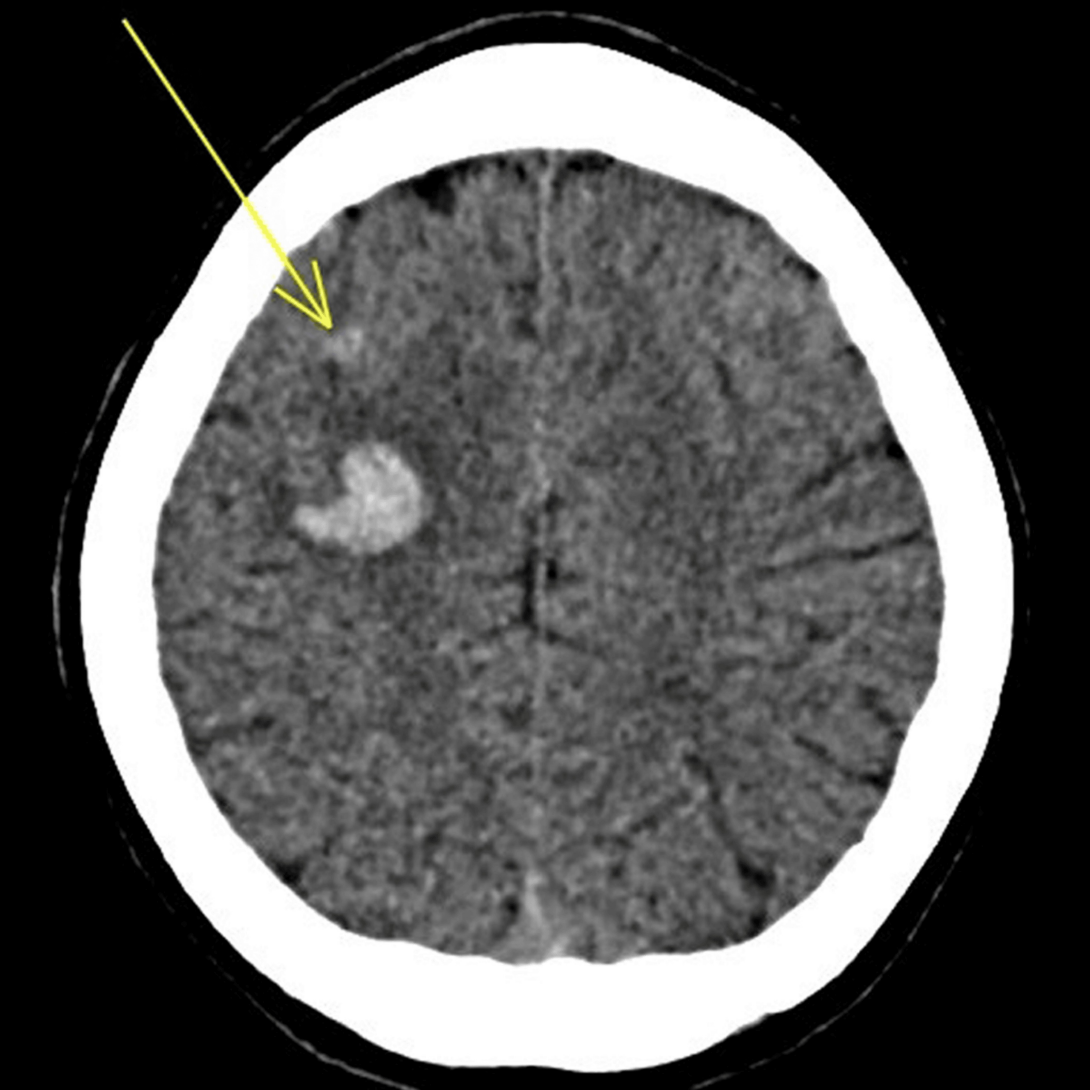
Axial CT head imaging showing a small area of brain micro-haemorrhage, highlighted by the yellow arrow
